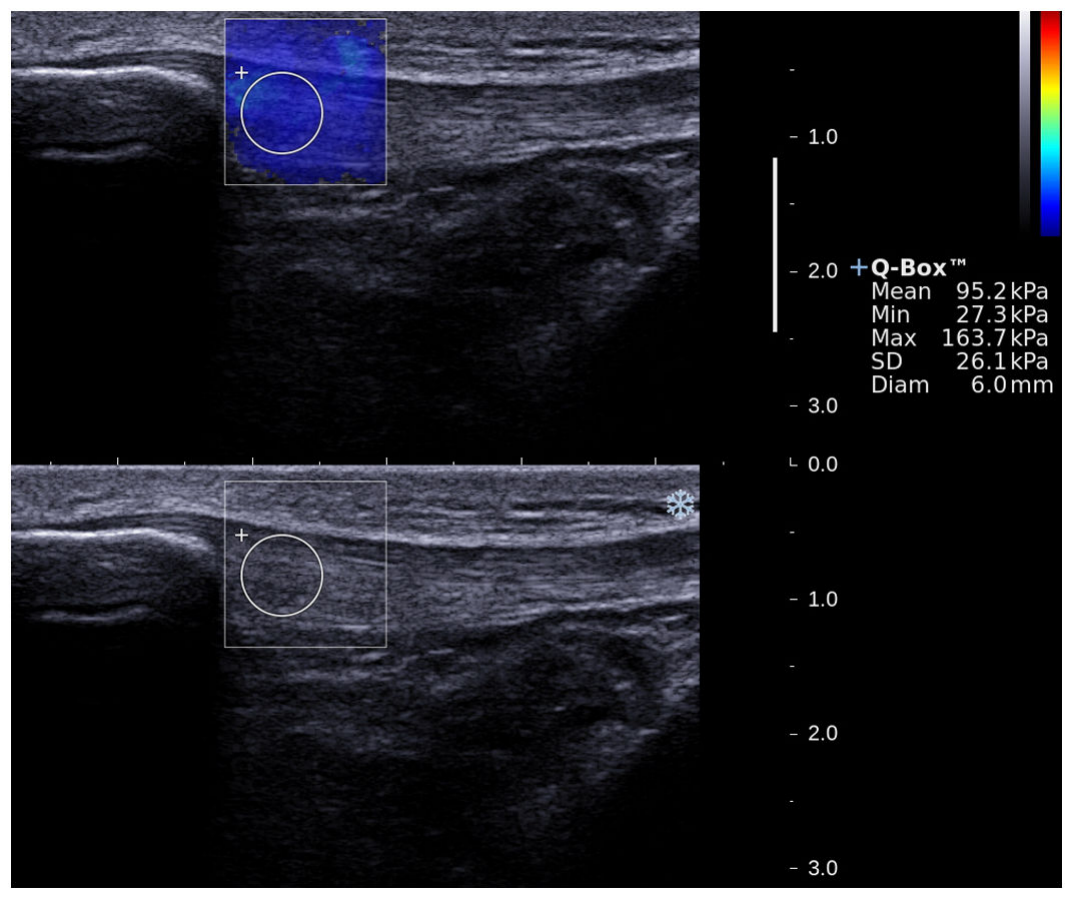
Typical example of elastic modulus measurement for the proximal patellar tendon on a healthy subject. Upper images show the color-coded box presentation of the PT elasticity (the red color represents the stiffer area and the green color represents the softer area) with the measurement circle representing the region of interest and its corresponding elastic modulus demonstrating under Q-Box^TM^ on the right. The transducer was kept motionless for 8 to 12 seconds during the acquisition of the SSI sonogram and the diameter of the measurement circle was defined by the thickness of the tendon. The bottom images show the longitudinal grey scale sonograms of the PT to ensure the capture of clear images.
